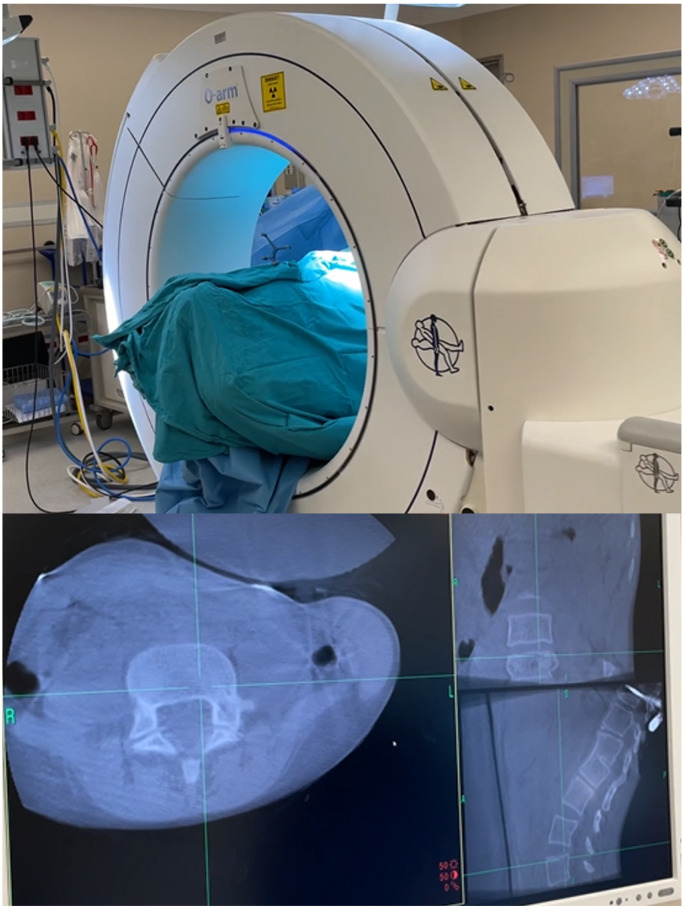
With the O-arm, a CT scan of the relevant area is taken. Planning can be done based on the CT images.
